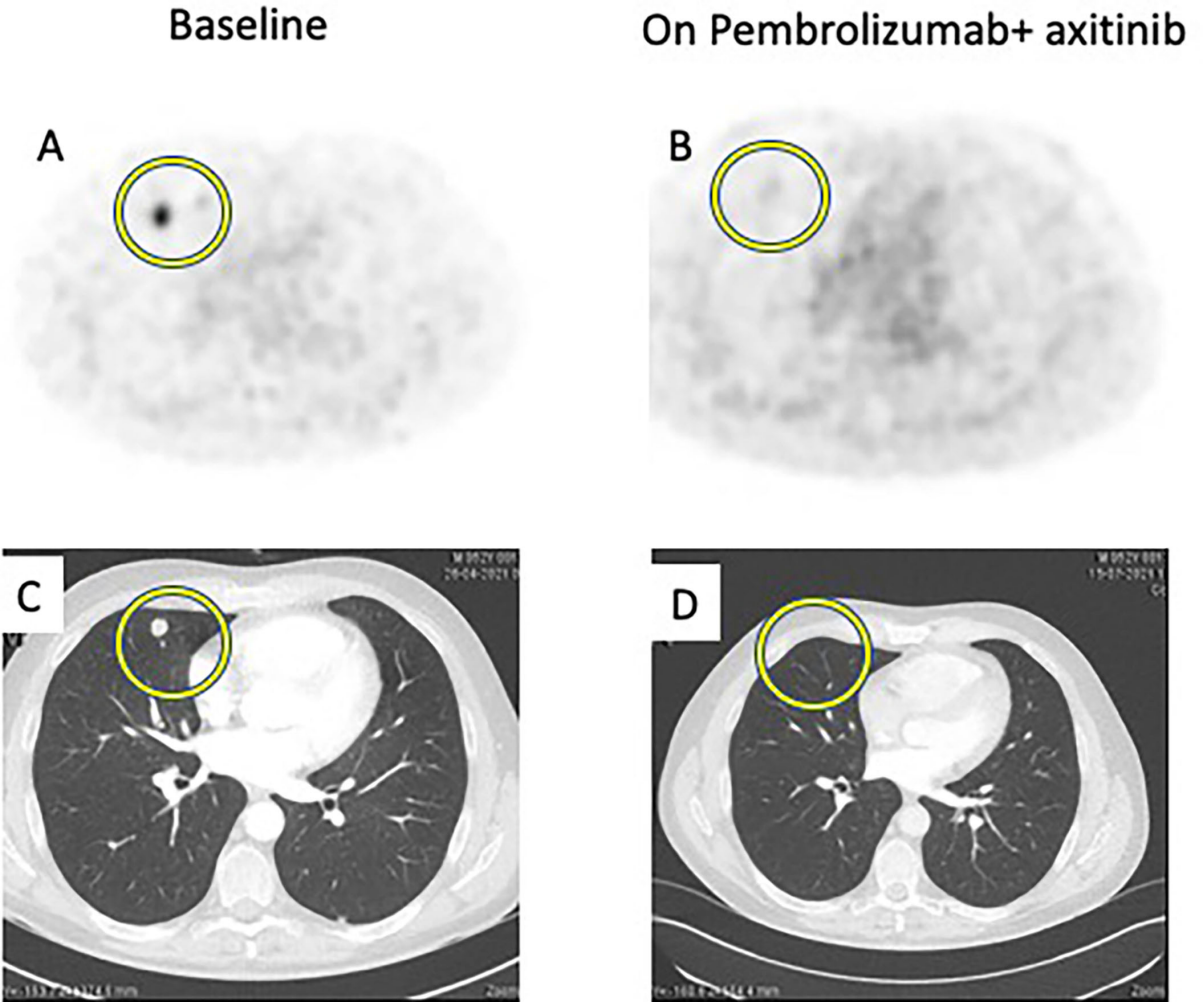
Comparison between baseline imaging and on-treatment imaging. ^68^GaPSMA-PET at baseline (A) and at 8-week (B) showing decrease of metabolism (yellow circle). Thoracic CT Scan at baseline (C) and at 12-week (D) showing disappearance of pulmonary lesions (yellow circle).
